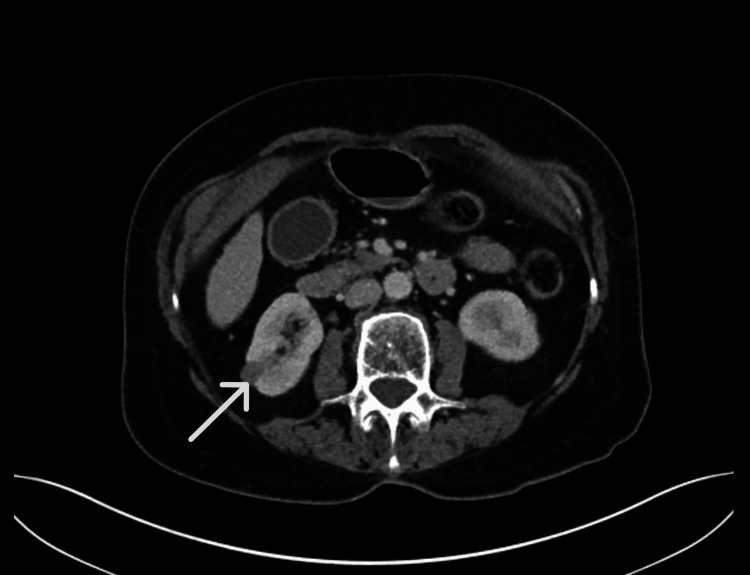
Axial CT renal Axial CT renal demonstrating a right-sided renal lesion (indicated by the arrow).
